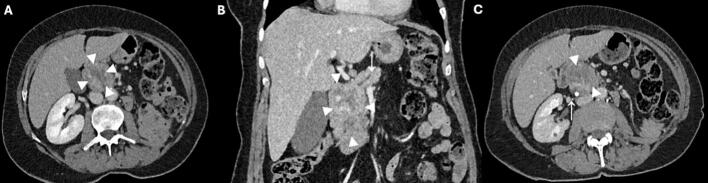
Portal venous phase abdominal CT in axial (A, C) and coronal (B) direction, showing a mass (between arrowheads) in the pancreatic head with a maximum diameter of 37 mm and heterogeneous enhancement. Also, the pancreatic duct proximal to the mass is dilated (arrow in image B). (C) showing the mass (between arrowheads) in the pancreatic head with a maximum diameter of 43 mm and heterogeneous enhancement. A stent in the common bile duct is present (arrow).
